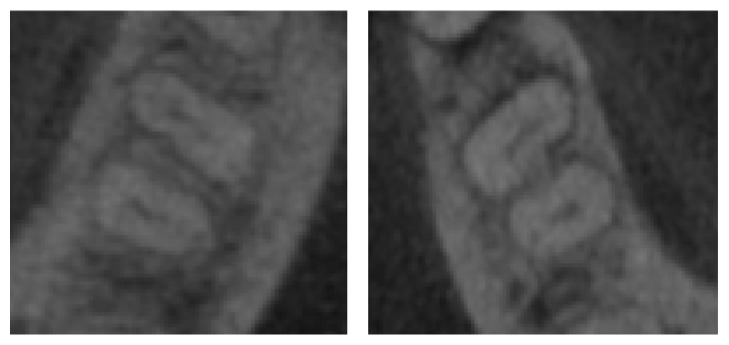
The examples of teeth images. The difference in the brightness between teeth and sockets of teeth in dental CT images is relatively low.
